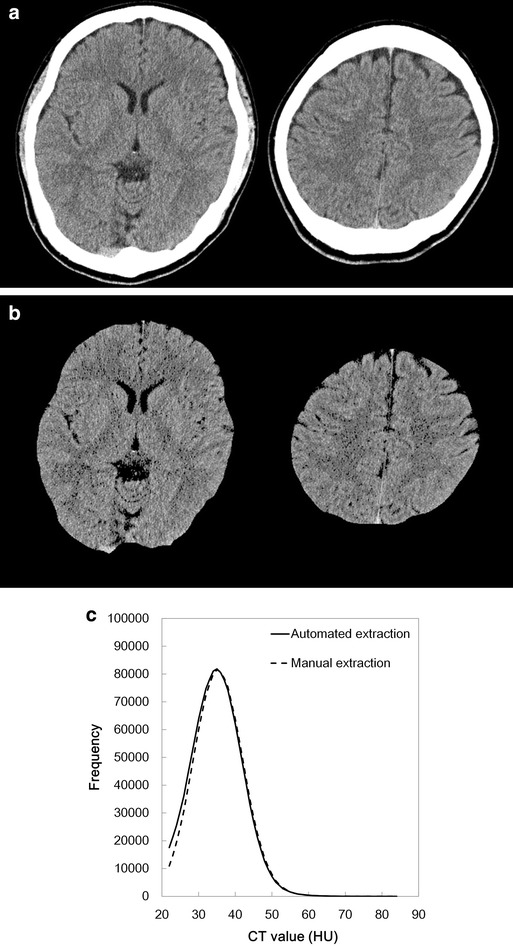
Representative CT images (a), extracted results from the corresponding slices (b), and CT histogram (c; *solid line*: automated extraction; *dashed line*: manual extraction) from a 27-year-old male. The similarity index was 0.937, which was the median of all the databases
